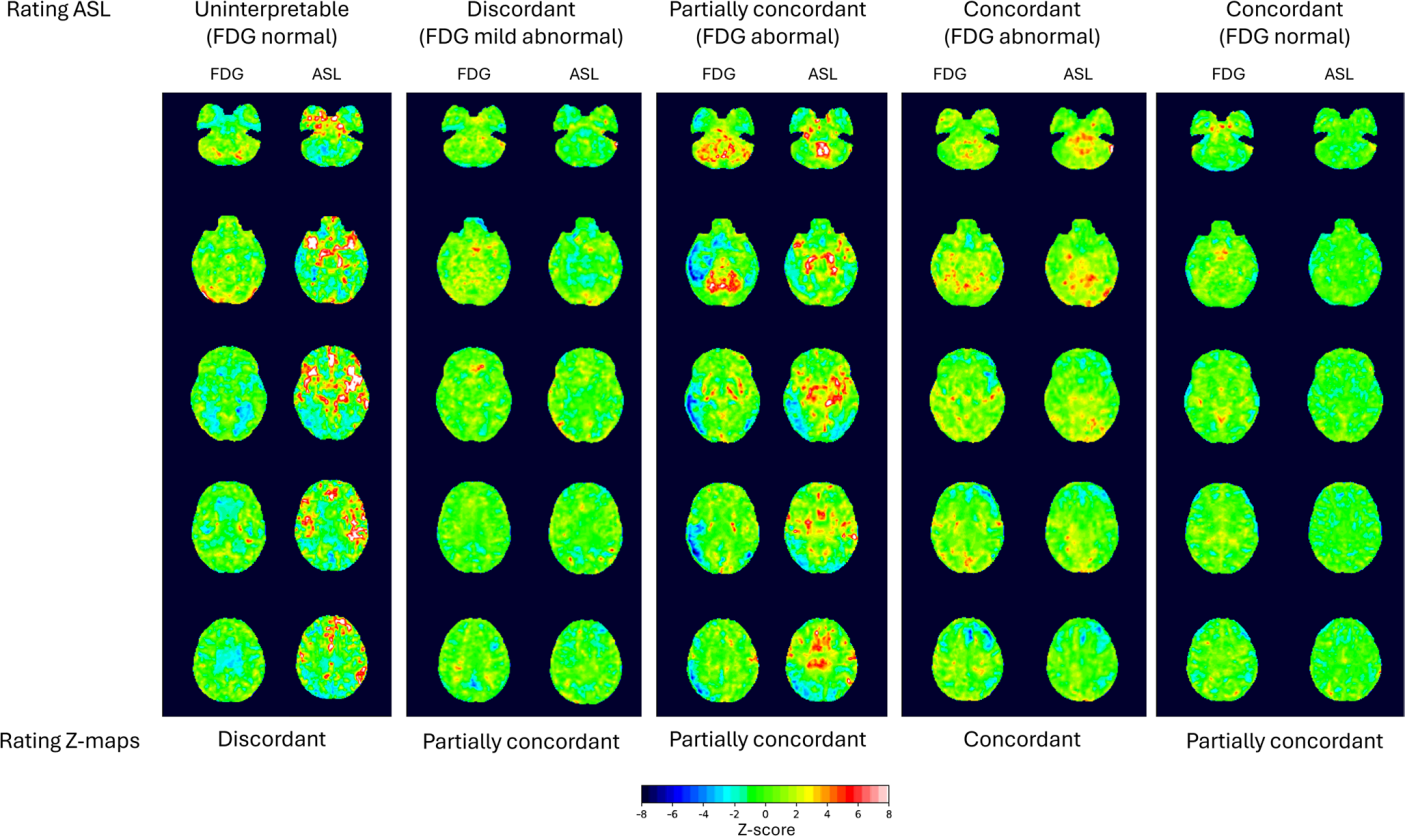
Examples of Z-score maps. Comparison of [^18^F]FDG PET and ASL Z-score based parameter maps. Five axial slices are shown for same subjects as in Fig. 1. Note areas of positive Z-scores (yellow/red) as a result of normalisation to whole cortex mean. Areas of negative Z-scores (blue) tends to be larger in [^18^F]FDG PET maps than ASL indicating higher sensitivity
